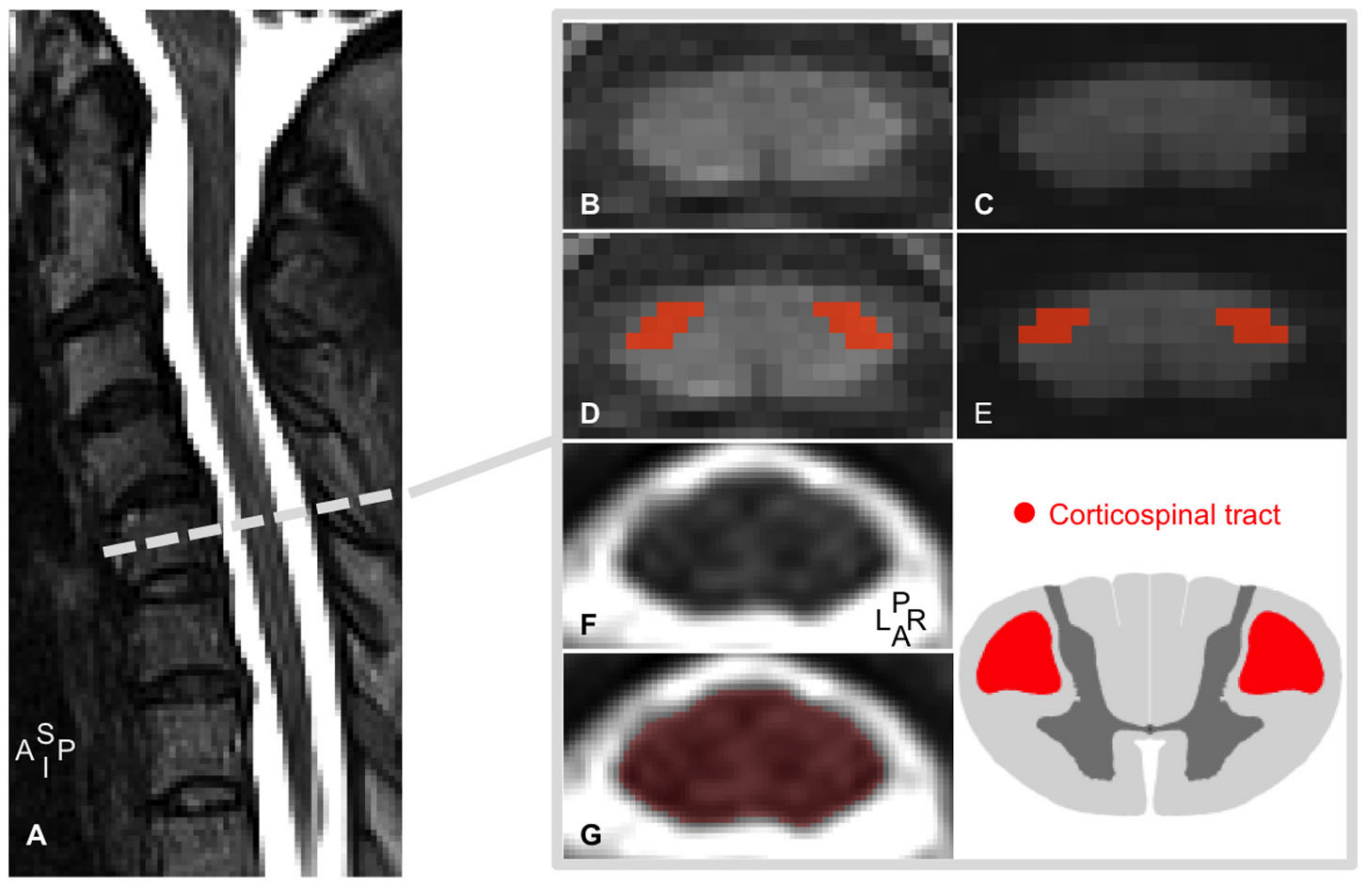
Regions of interest definition. (A) T2-weighted MRI mid-sagittal slice for an ALS patient. (B,C) T1-weighted data for MTR (B) and mean diffusion-weighted data for DTI (C). (D,E) Defined anatomical ROIs. ROIs were selected in the dorso-lateral (red) aspect of the spinal cord to include most of the CST. (F,G) T2-wheighted data before (F) and after (G) cord outlining. A, anterior, I, inferior; L, left; R, right; P, posterior; S, Superior.
